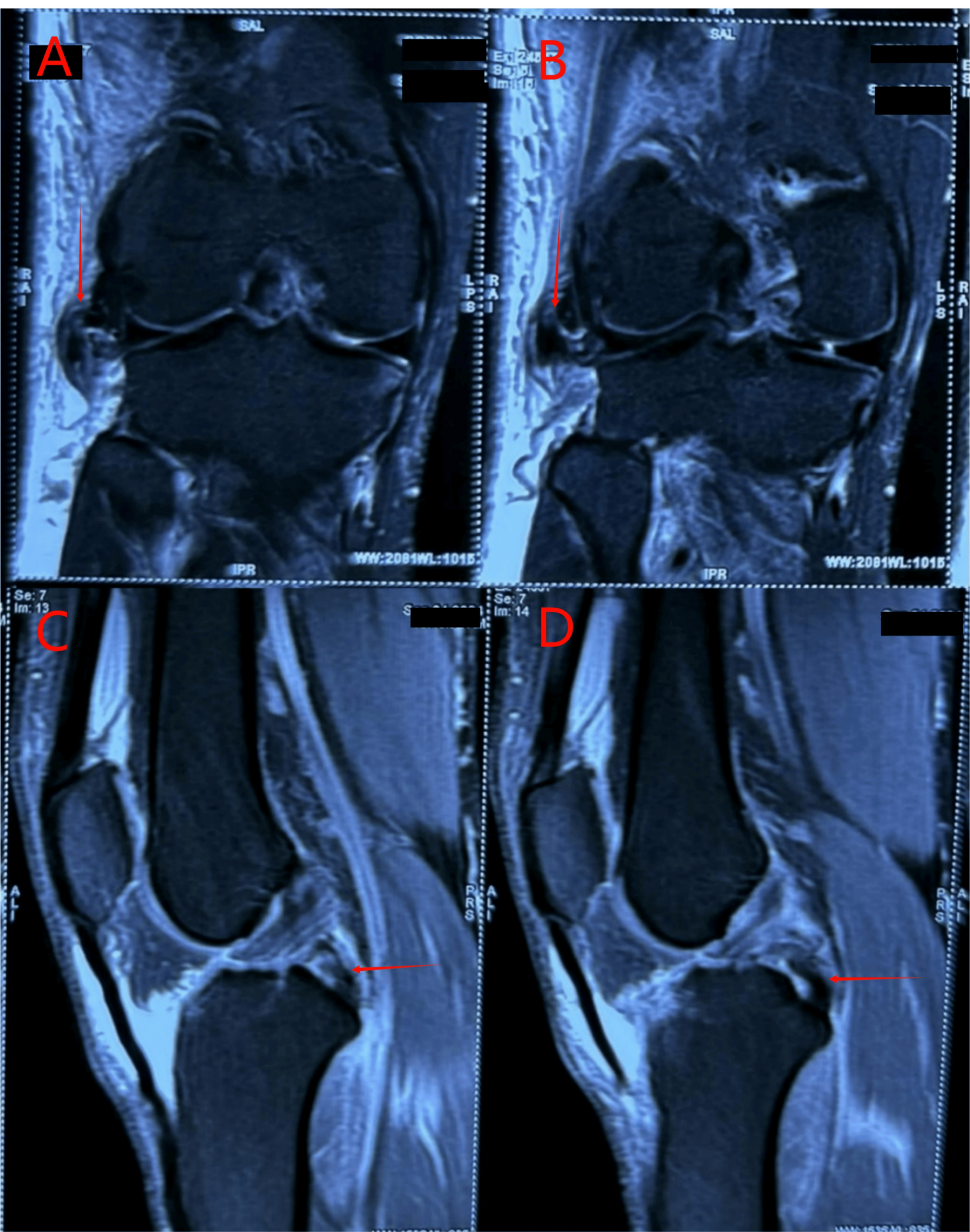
MRI of right knee (two coronal and two sagittal views) (A and B) PLC avulsion injury showing the lack of continuity in the fibers of the lateral collateral ligament in coronal views. (C and D) PCL tear showing the lack of continuity of PCL fibers in sagittal views MRI, magnetic resonance imaging; PLC, posterolateral corner; PCL, posterior cruciate ligament
